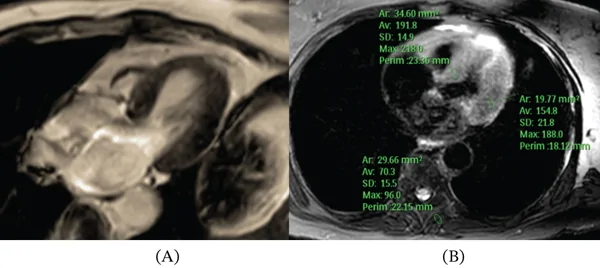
Cardiac MRI showing (A) aortic outflow long axis plane demonstrating patchy, mid‐myocardial delayed enhancement predominantly in the mid to distal septum and anterior wall and (B) edema sequence comparing myocardium signal intensity with skeletal muscle.
